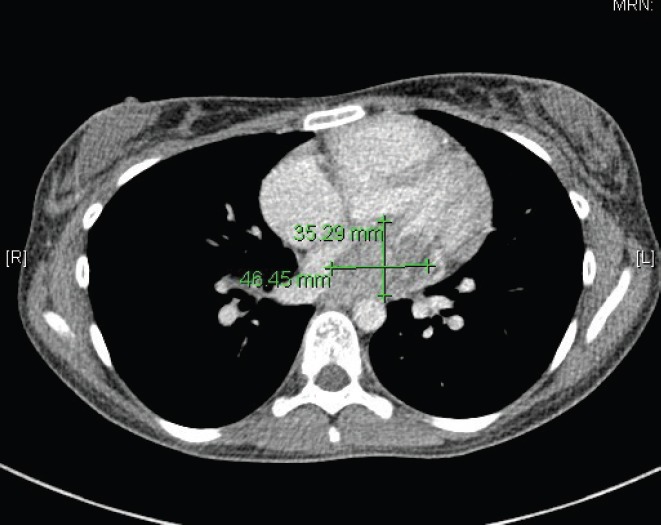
2015 CT thorax with contrast.
